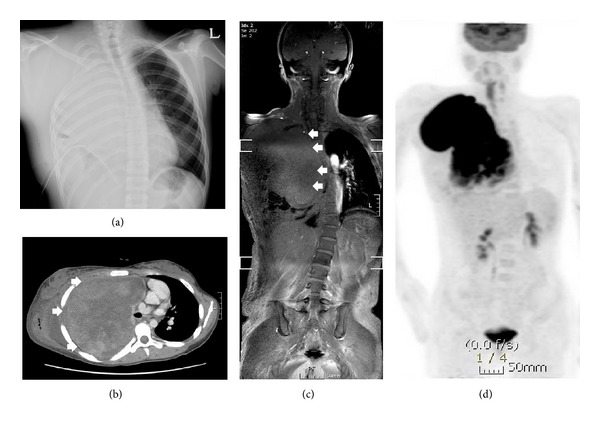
The patient's chest radiography shows a large thoracic mass with total collapse of right lung (a). Her chest CT scan (b) and spine MR imaging (c) also show a large intrathoracic hyperdense mass (white arrows) in the right chest, resulting in prominent scoliosis and cardiac deviation. Her ^18^F-FDG PET/CT scan showed highly increased FDG uptake (SUV_max⁡_ = 6.9) in this mass, suggesting highly malignant tumor (d).
